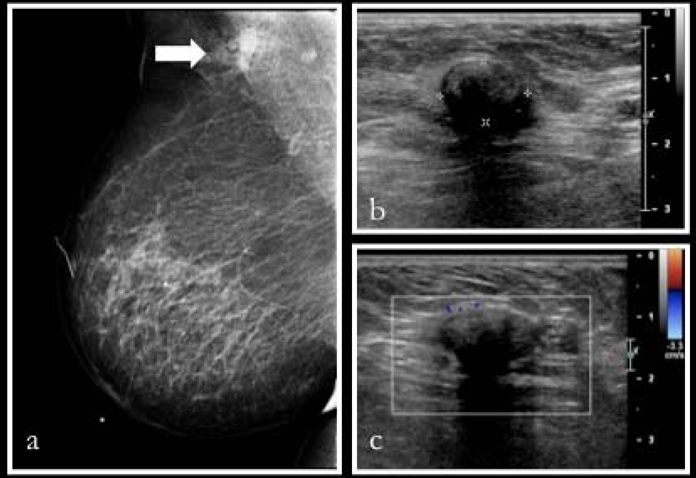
(a) Right medio-lateral oblique mammogram showed an ill-defined high density mass in the right axilla (white arrow). (b, c) Ultrasound of the axilla with a solitary well-defined hypoechoic lesion with acoustic shadowing and absence of vascular flow, appearing similar to a lymph node.
